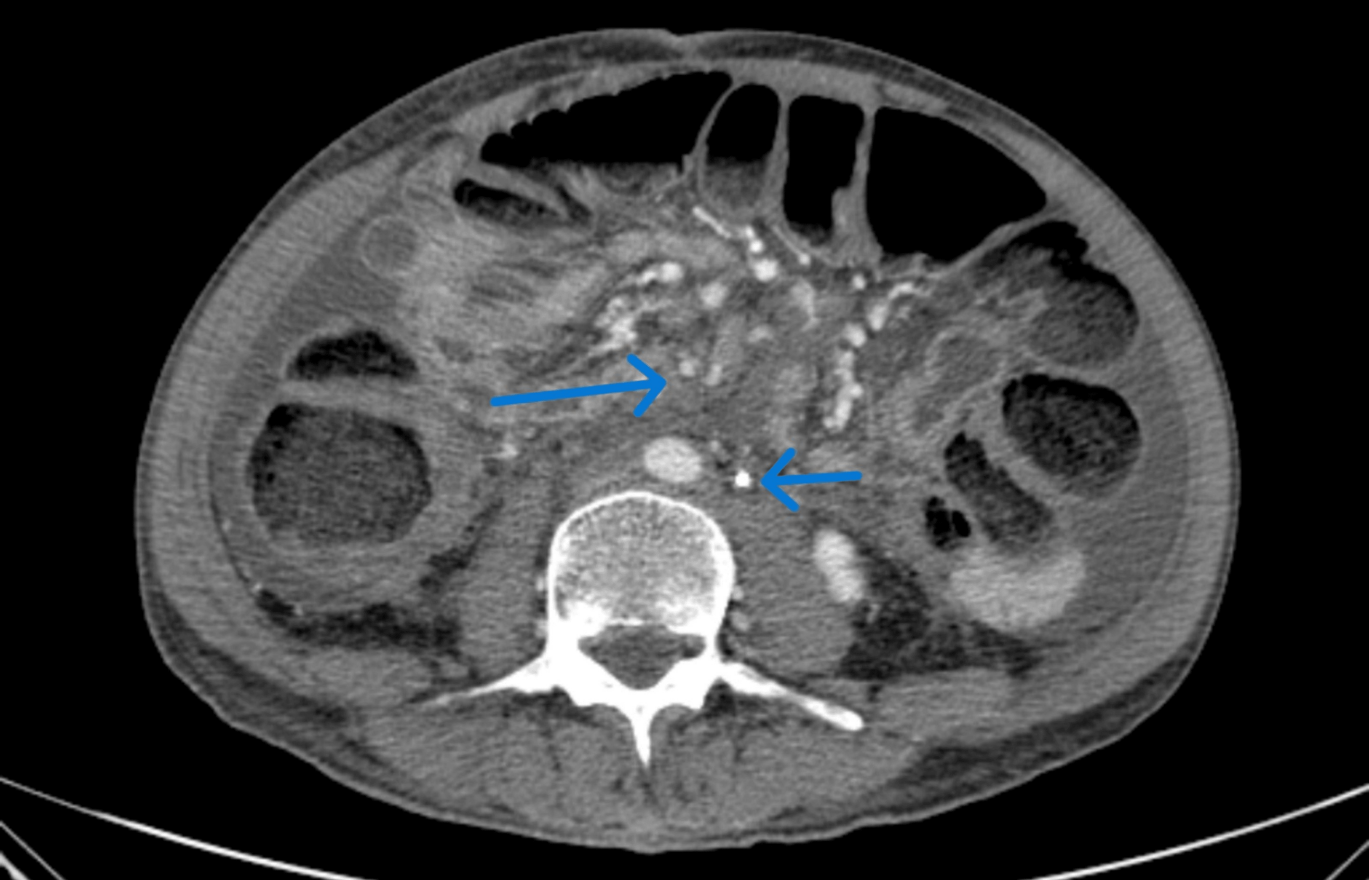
Axial contrast-enhanced CT scan showing retroperitoneal soft tissue encasing the abdominal aorta and bilateral ureters, consistent with retroperitoneal fibrosis.
